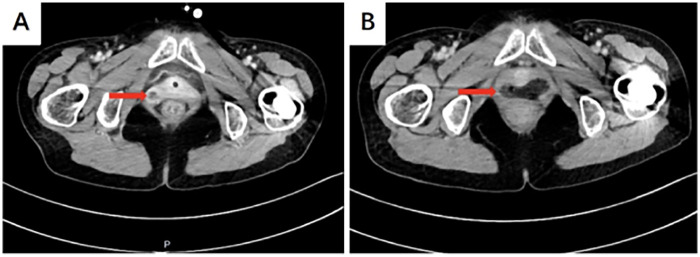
Whole abdomen + pelvis CT: (A) a mass is seen at the junction of the middle and lower 1/3 of the right side wall of the vagina, with a maximum diameter of about 22 mm (red arrow); (B) no obvious abnormality is seen in the vaginal wall: (red arrow).
